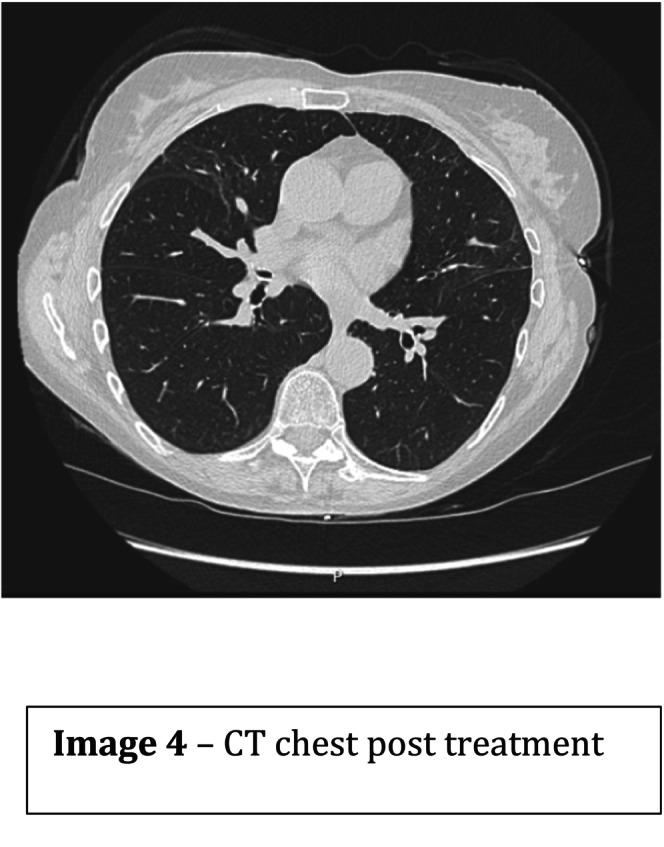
CT chest post treatment.
